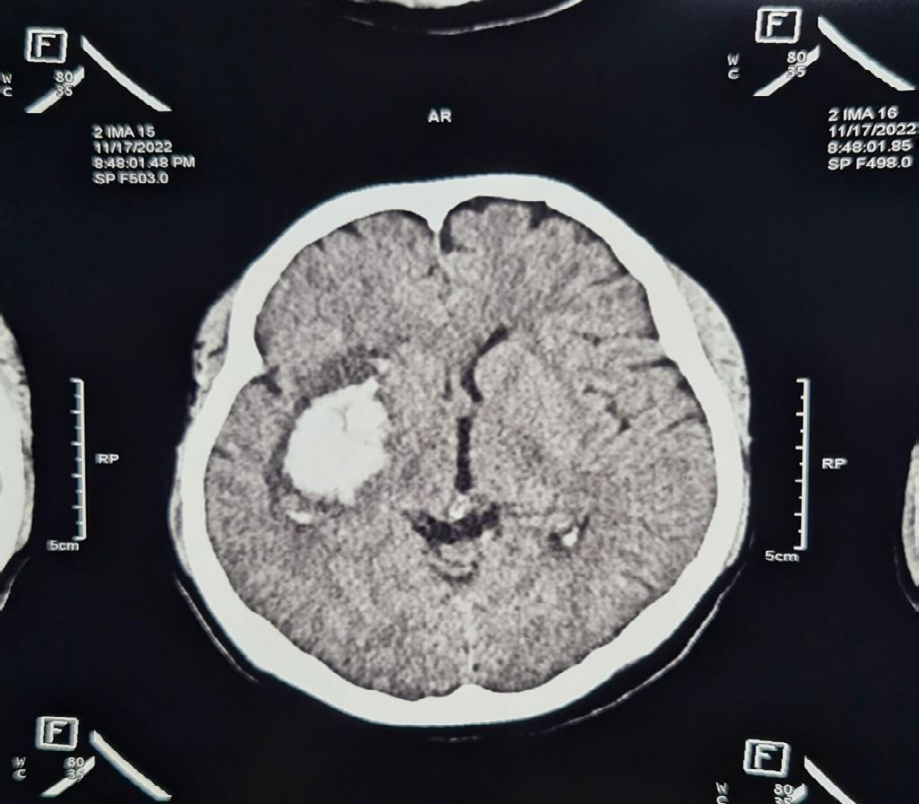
Noncontrast computed tomography head - Intracranial Hemorrhage.
